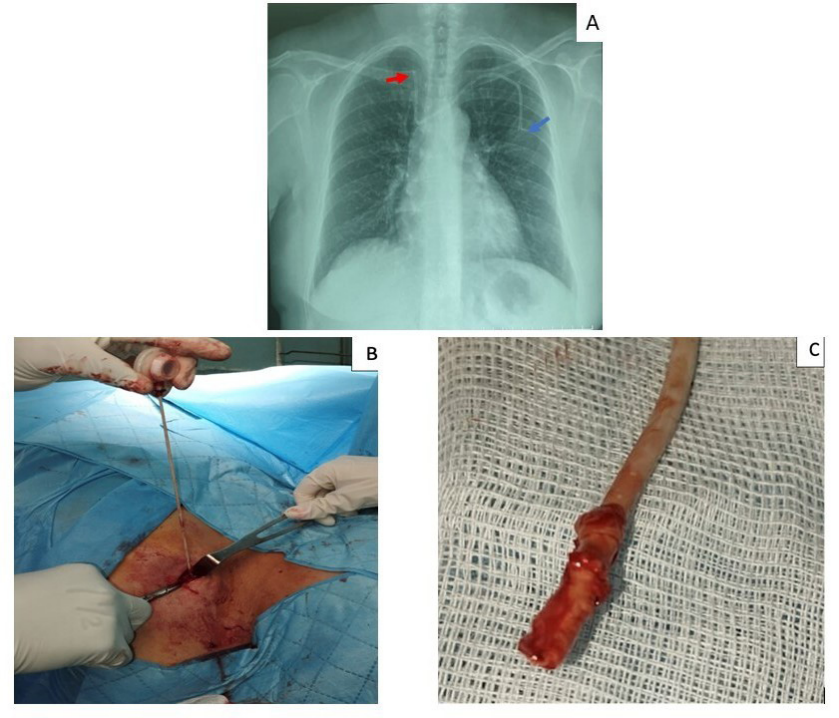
(A) Chest radiograph showing an aberrant course of the Port-a-Cath, with the distal end lodged in the right brachiocephalic vein (red arrow) and a slightly visible port chamber (blue arrow); (B) Traction of the polyurethane catheter, which was held in place by distal adhesion; (C) Fibrotic encapsulation in the distal end of the catheter.
